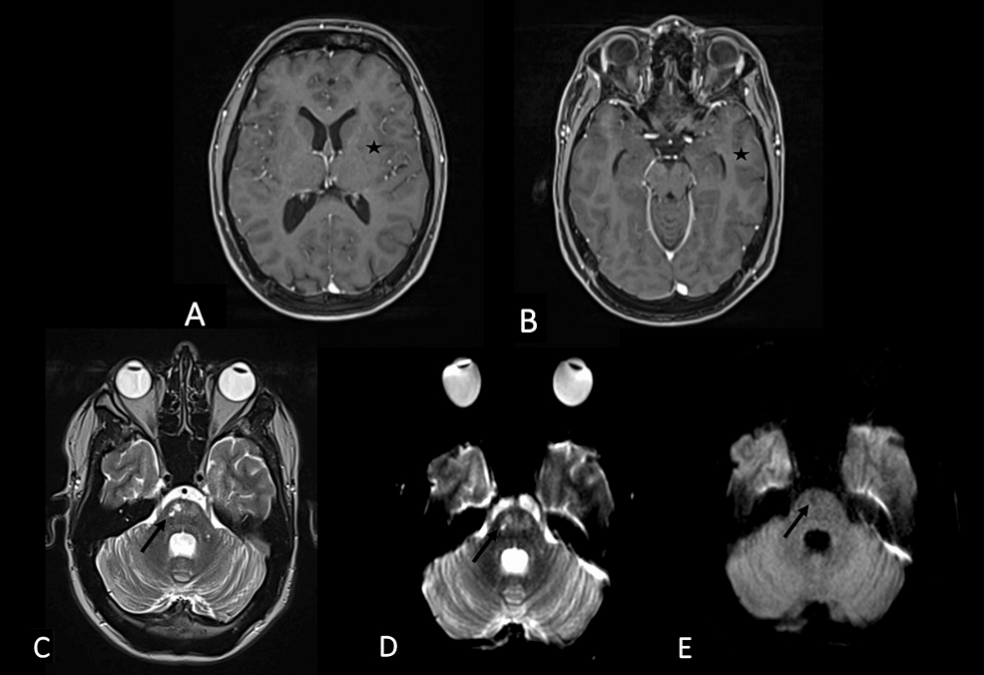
Brain MRI after three months of treatment MRI showing complete resolution of the leptomeningeal nodular enhancement after treatment (black stars) displayed on post-contrast axial T1WI (A,B). Pontic and brain stem chronic ischemic lesions are demonstrated (black arrows), characterized by a hypersignal on sequences axial T2 (C) without diffusion restriction (D,E). MRI: magnetic resonance imaging, T1WI: T1-weighted image
